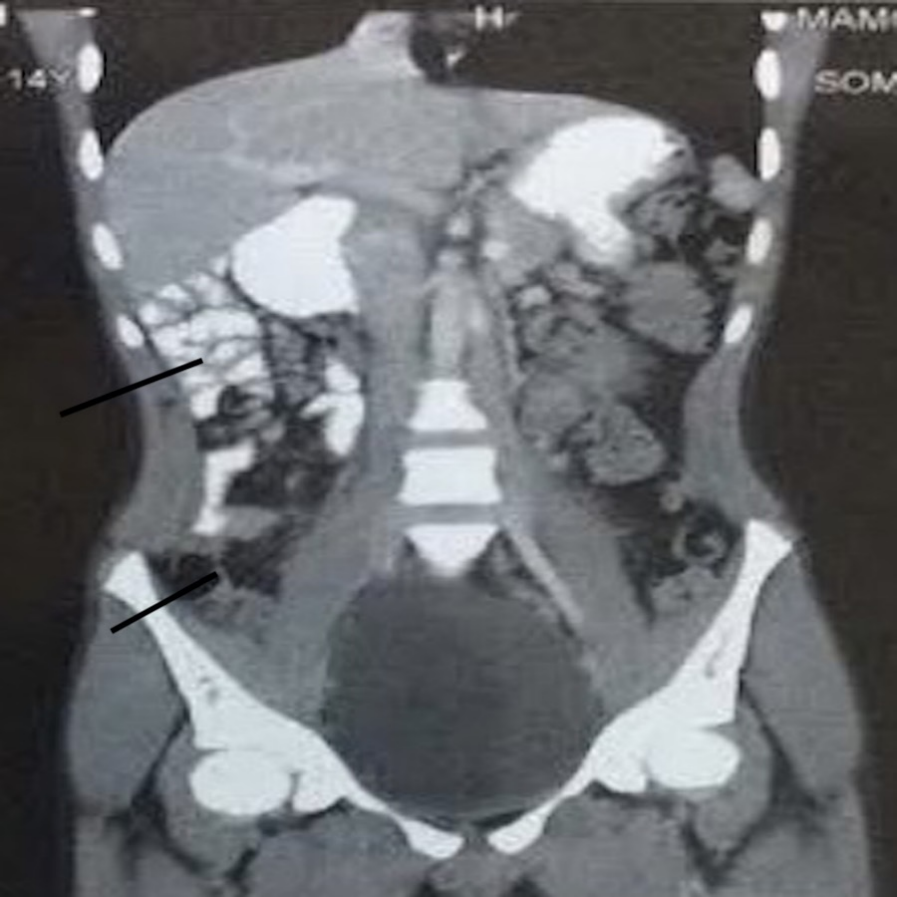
CT showing small bowel loops on the right side and lack of visualization of the caecum in the right iliac fossa CT: computed tomography
